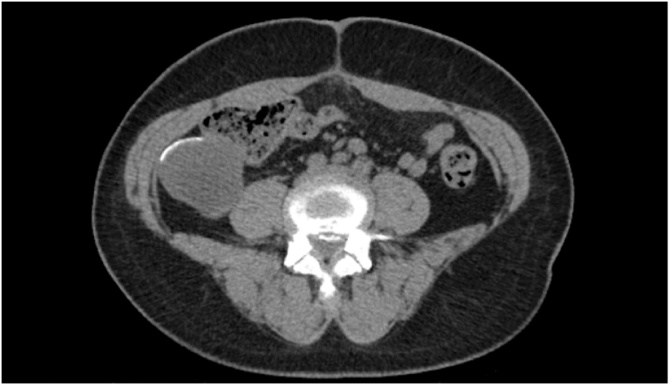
Computed tomography of the abdomen and pelvis demonstrating a 7.6 × 4 cm mass with peripheral calcification suspicious for a mucocele versus mucoid epidermoid carcinoma.
